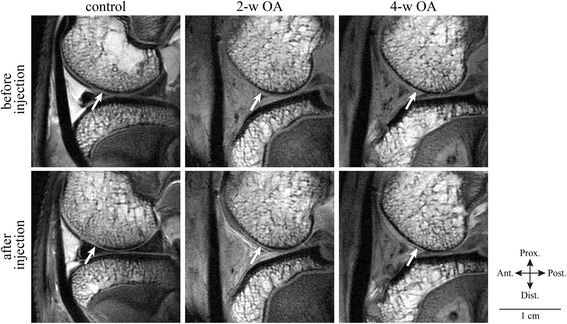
MR images from the present study. Representative proton density-weighted images are shown. The top row shows MR images of the control, the 2-w OA and 4-w OA before contrast agent injection, and the bottom row after contrast agent injection. The arrows indicate the surface of the cartilage in the control, 2-w OA and 4-w OA. Scale bar, 1 cm. Ant. = anterior, Post. = posterior, Prox. = proximal, and Dist. = distal
